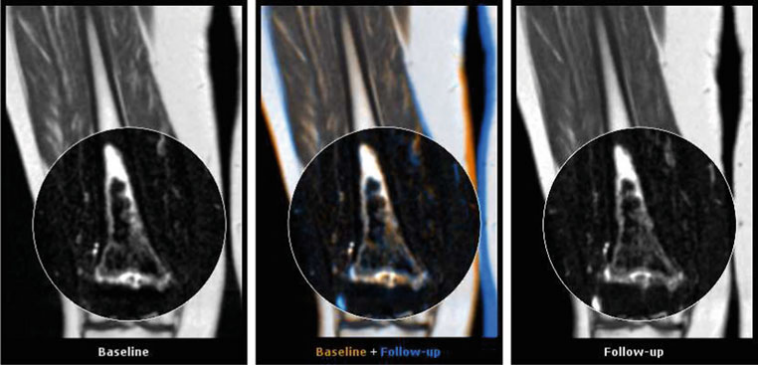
Slice view of the data, with the STIR lens overlay enabled. From *left* to *right*: baseline, color fusion of baseline and follow-up, and follow-up. The white circular lens, which follows the cursor, shows a secondary MRI modality, providing better definition of the cancerous lesions. The image shown inside is co-located with the surrounding data, which as such functions as an anatomical reference. The STIR lens also participates in the color fusion, so that changes in intensity in the secondary data are easily visualized
